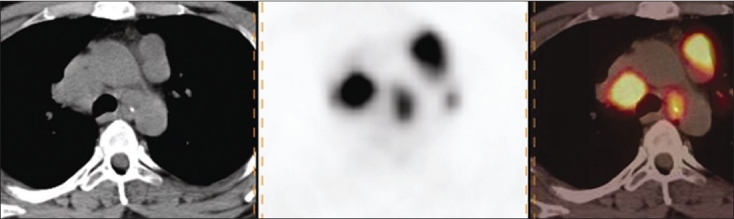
Axial CT, PET, and PET-CT images showing increased FDG uptake in mediastinal lymph nodes in a patient having sarcoidosis
